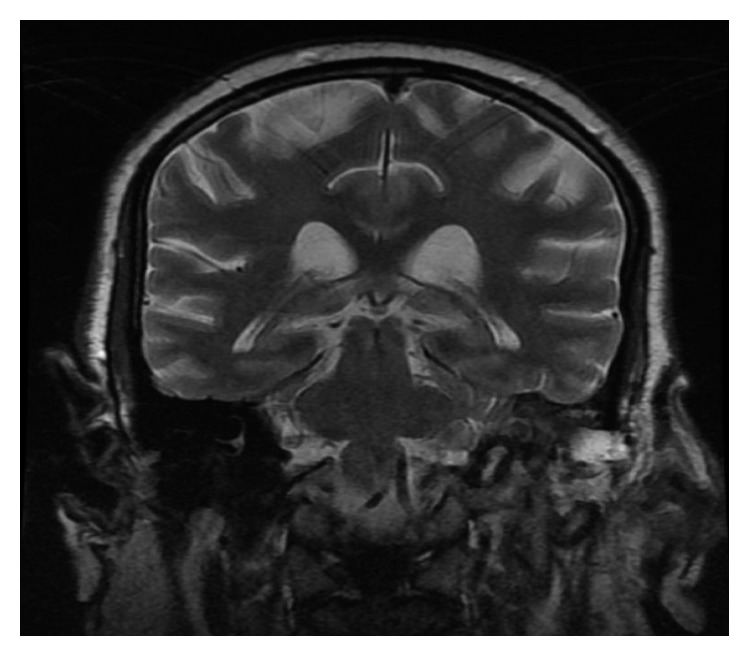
MRI of cerebellopontine angles and posterior fossa (coronal cut). Opacification of the left mastoid air cells and middle ear cavity with soft tissue signal which elicits low signal in T1WI and high signal in T2WI with restricted diffusion in DWI. There is homogeneous enhancement following IV contrast administration. No evidence of adjacent intracranial abscess formation or sinus thrombosis. The region of brain stem and cerebellar hemispheres are normal. No focal lesions are seen. Both the VII and VIII cranial nerves show normal course and signal intensity. No intra- or extra-canalicular enhancing lesion is seen.
